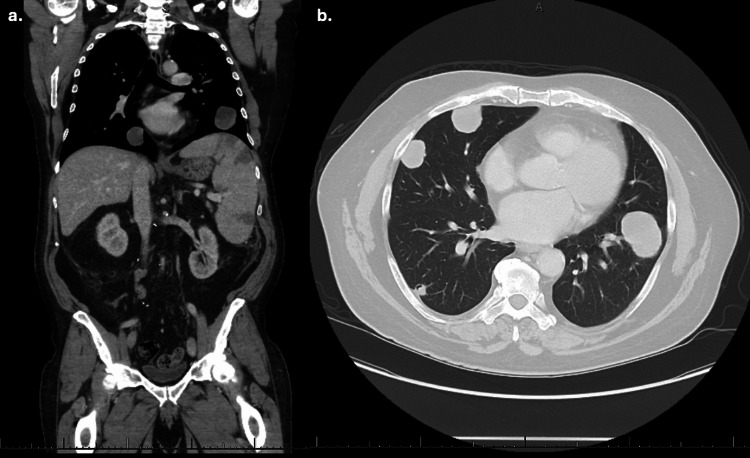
CT chest, abdomen, and pelvis with intravenous contrast in the coronal (a) and transverse (b) plain showing paraesophageal nodules, multiple pulmonary nodules, and splenomegaly with new splenic infarcts.
